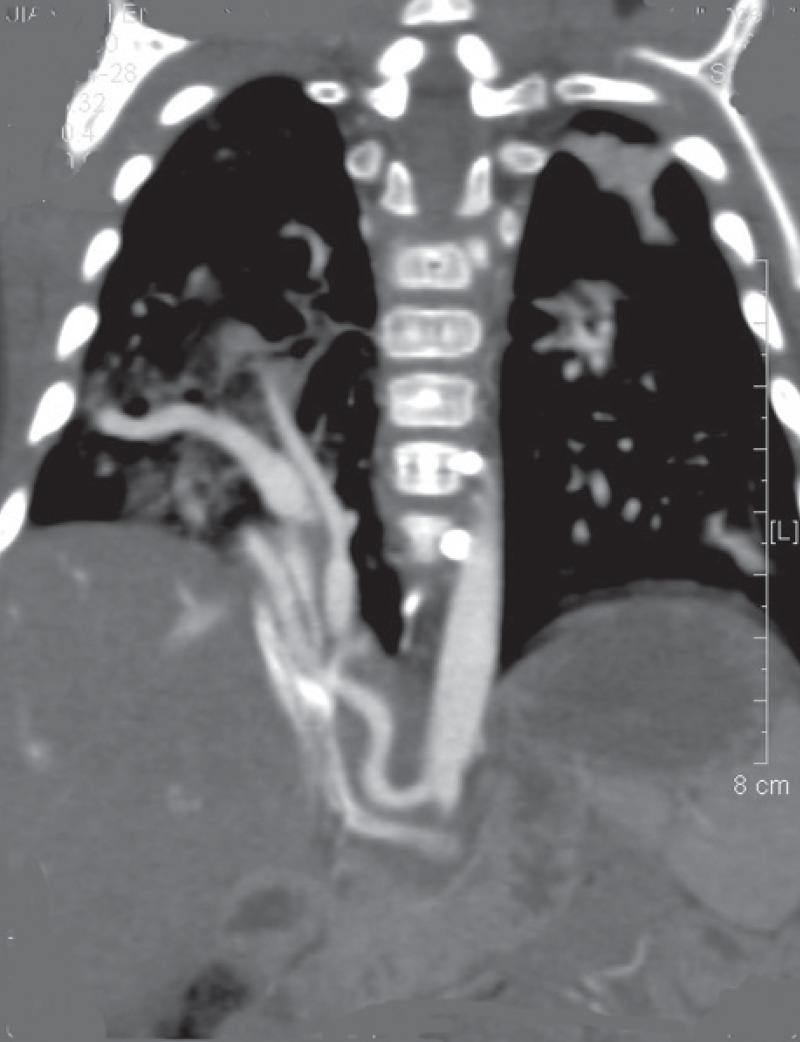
CTA showing right pulmonary vein drainage to the inferior vena cava.
